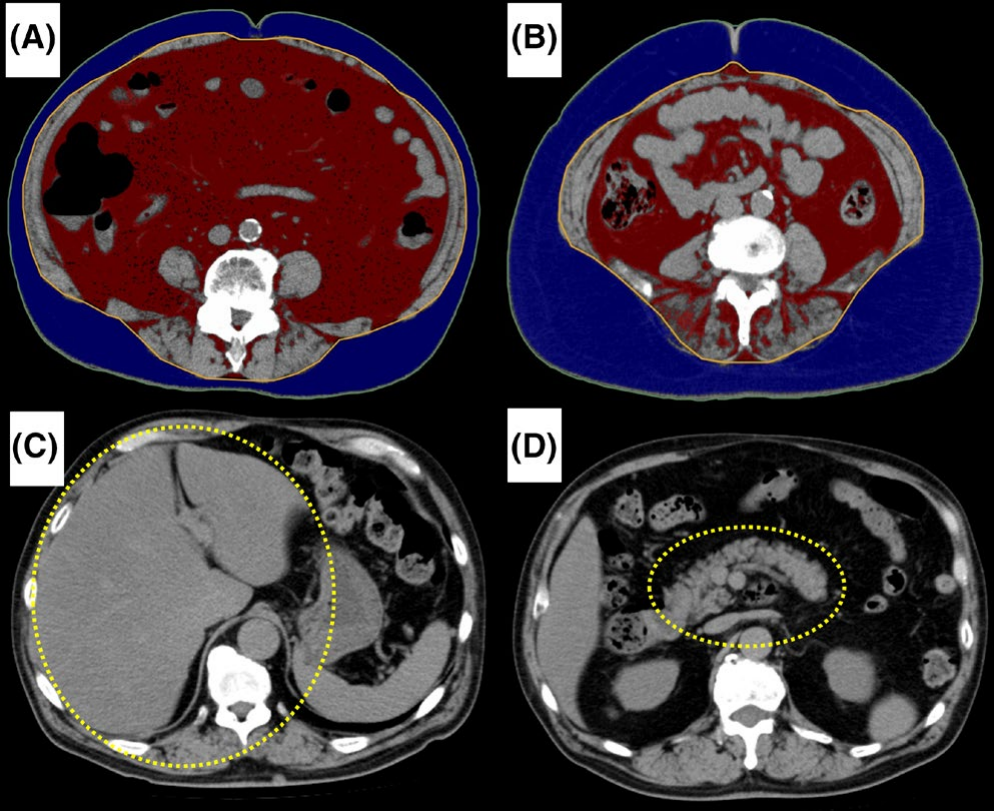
Analysis of visceral fat areas, subcutaneous fat areas, fatty liver, and pancreatic fatty replacement. Computed tomography (CT) imaging analysis at the umbilicus level in the axial plane: Red areas indicate visceral fat areas (VFAs), whereas blue areas indicate subcutaneous fat areas (SFAs). The presence of fatty liver and pancreatic fatty replacement was verified via abdominal computed tomography (CT) images, highlighted in yellow circles. (A) Patient with high VFA. (B) Patient with high SFA. (C) Patient with a fatty liver. (D) Pancreatic fatty replacement.
